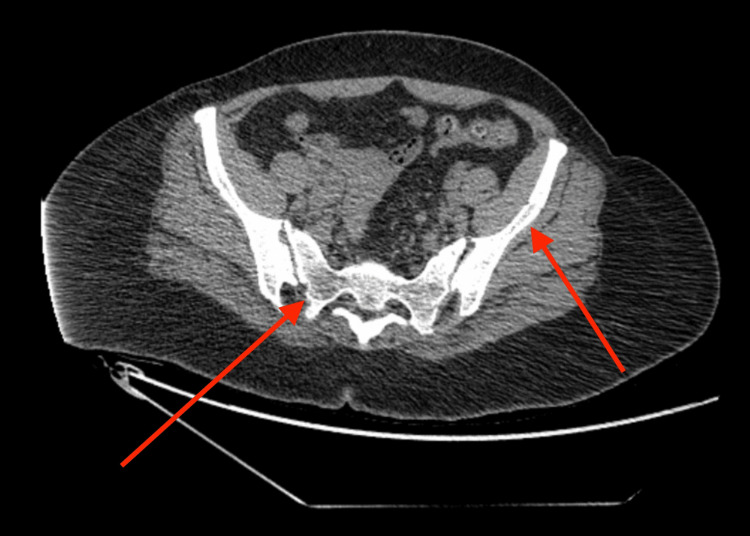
CT of the abdomen and pelvis showing bilateral iliac lymphadenopathy and erosive lesions of the spine and iliac bones The red arrows show erosive lesions CT: computed tomography
